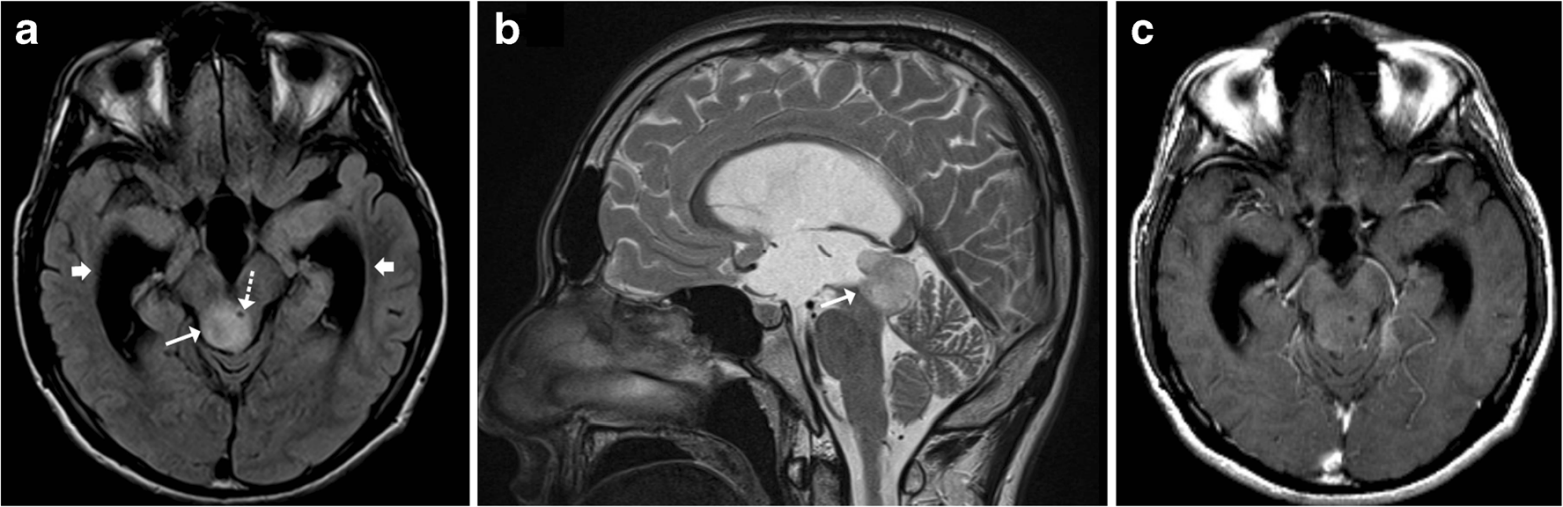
A 16-year-old male with headache and vomiting: a–b Axial FLAIR and T2-weighted sagittal MRI shows a relatively well-defined hyperintense lesion involving the tectum (*arrow*). The aqueduct is encased and compressed (*dotted arrow*) with resultant dilatation of the temporal horns (*block arrow*). c No significant contrast enhancement is noted in keeping with a glioma
